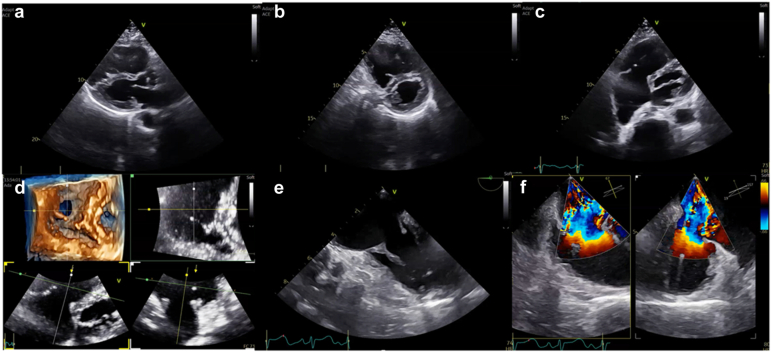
TTE and TEE evaluation of a patient with torrential TR related to a carcinoid disease. (a and b) Severe RV dilatation with diastolic septal shift of the interventricular septum. (c and d) Important tethering and restriction of the tricuspid leaflets and wide coaptation gap in 2D and 3D MPR. Significant thickening of the subvalvular apparatus was also observed in TTE (e) with torrential TR in color Doppler (f) Abbreviations: MPR, multiplanar reconstruction; RV, right ventricle; TEE, transesophageal echocardiography; TR, tricuspid regurgitation; TTE, transthoracic echocardiography.
